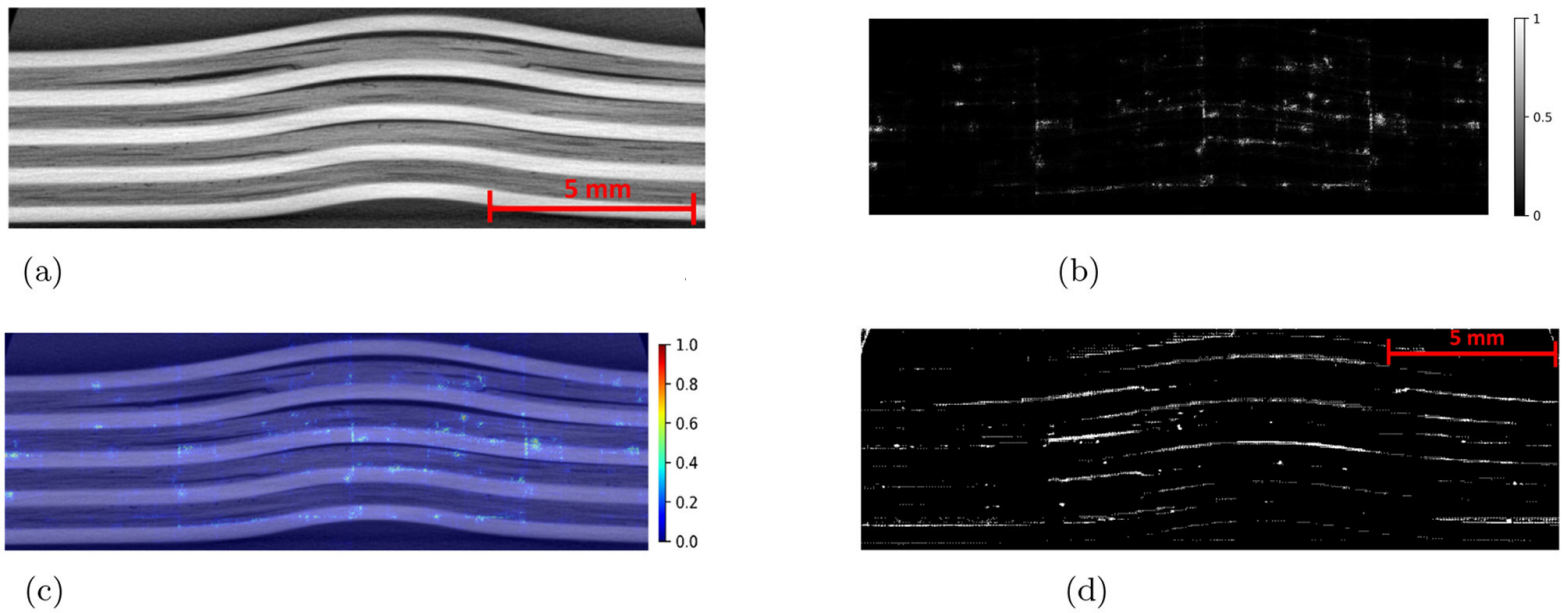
An example CT slice of the damaged plate with 10 Joules impact energy is shown in (a). The attribution map (b) is produced by applying the IG method to the trained SAM. The overlap of the attribution map and CT slice in (c) suggests that the SAM has very low interpretability. (a) CT slice with impact energy of 10 Joules. (b) Attribution map. (c) Overlap of attribution map and CT slice. (d) Predicted mask.
